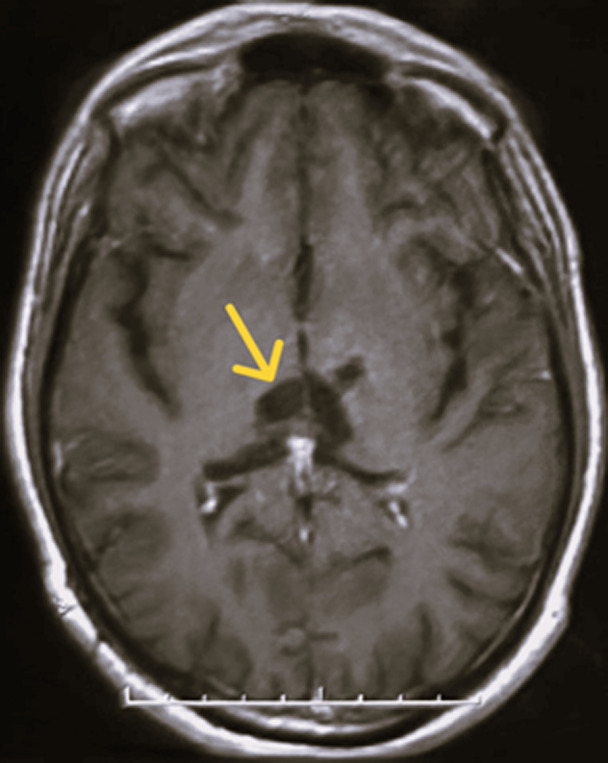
post-treatment contrast-enhanced brain magnetic resonance image showing regression of the thalamo-peduncular lesion: axial T1-weighted post-contrast magnetic resonance image obtained after treatment demonstrating cystic regression of the lesion with absence of residual contrast enhancement (yellow arrow)
